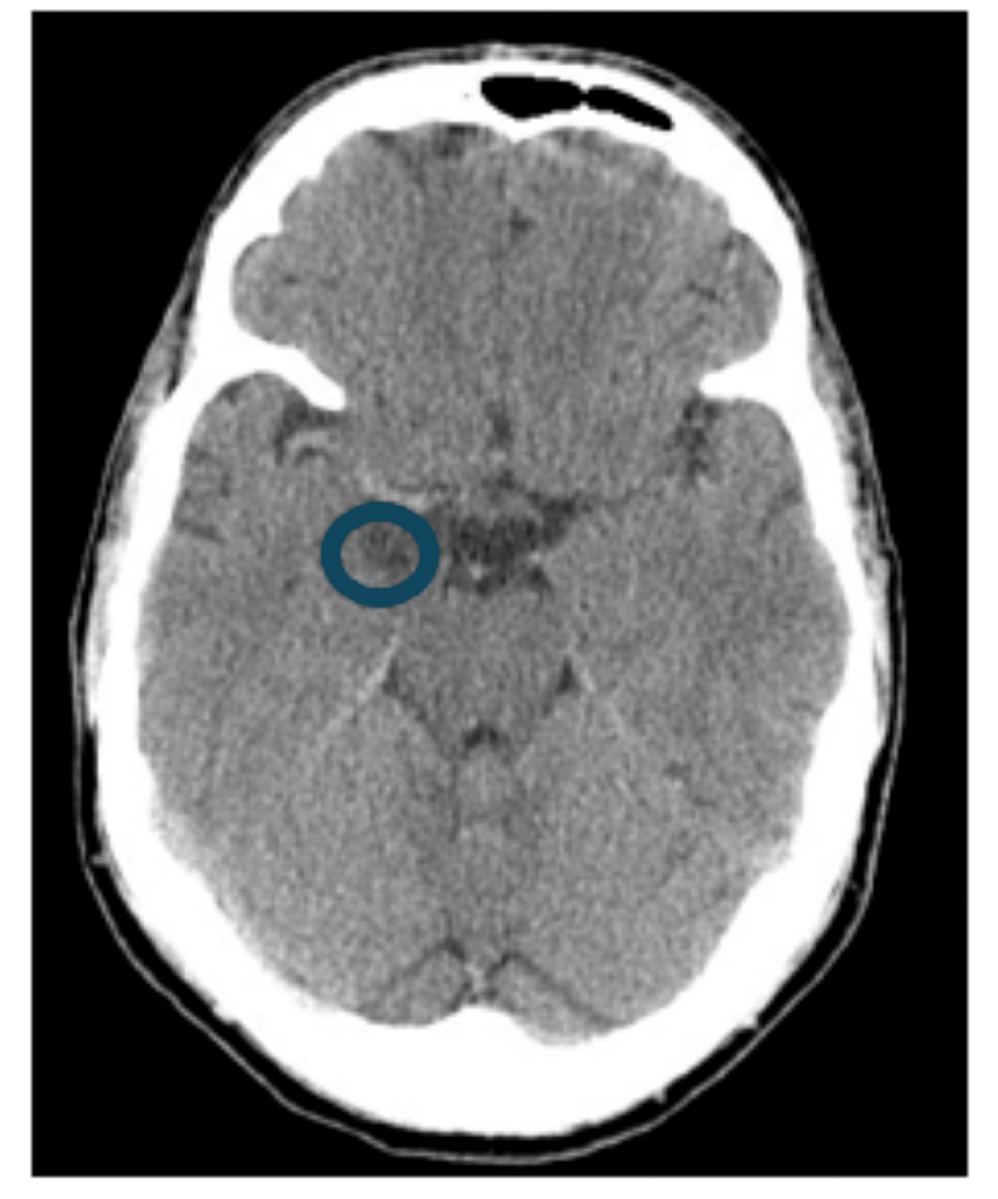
Axial CT image showing a hypodense lesion in the right mesial temporal region (blue circle).
